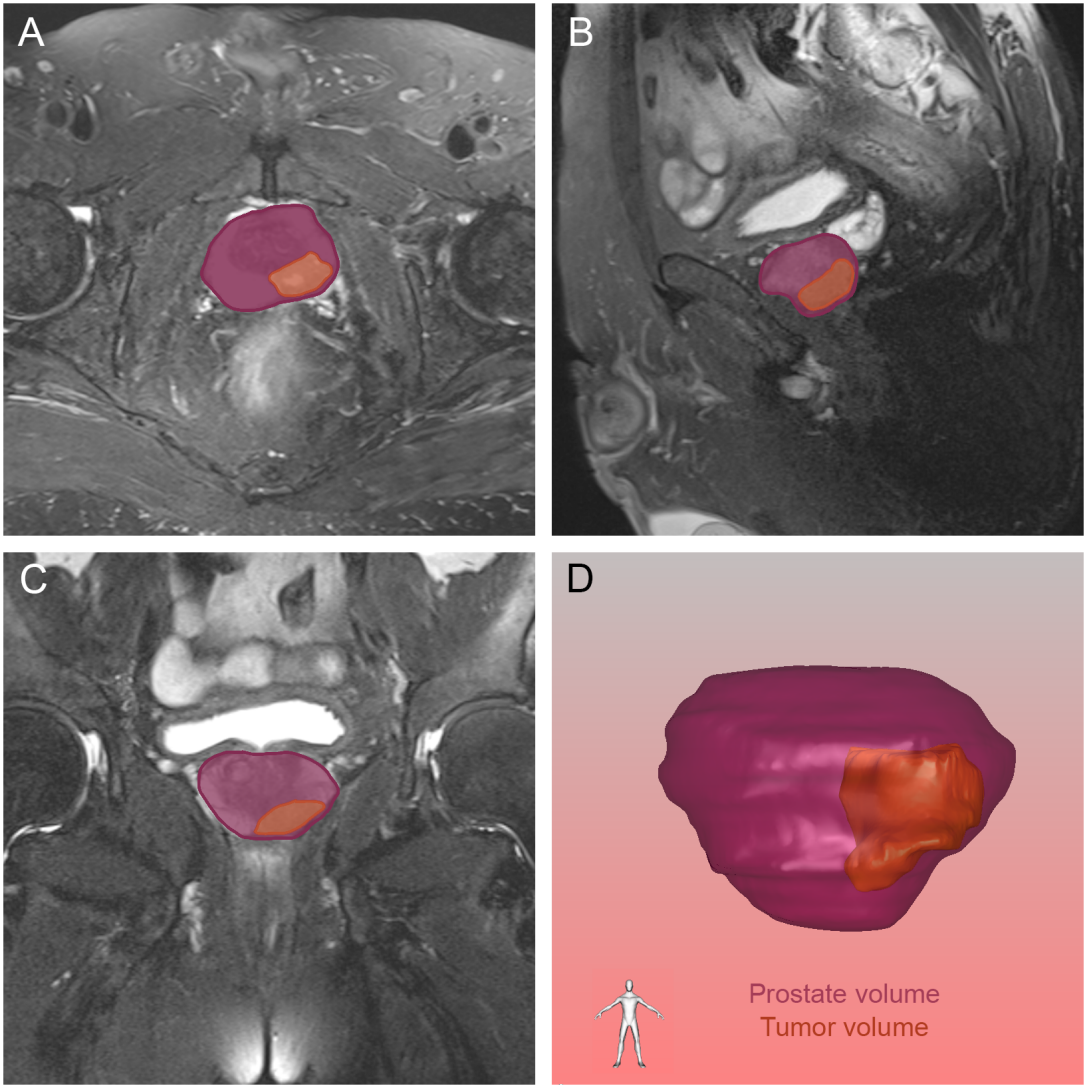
The tumor and prostate region in the axial (A), sagittal (B) and coronal (C) views on magnetic resonance imaging, and prostate volume and tumor volume reconstructed by three-dimensional visualization (D). The orange and purple sections indicated the prostate cancer and prostate respectively.
